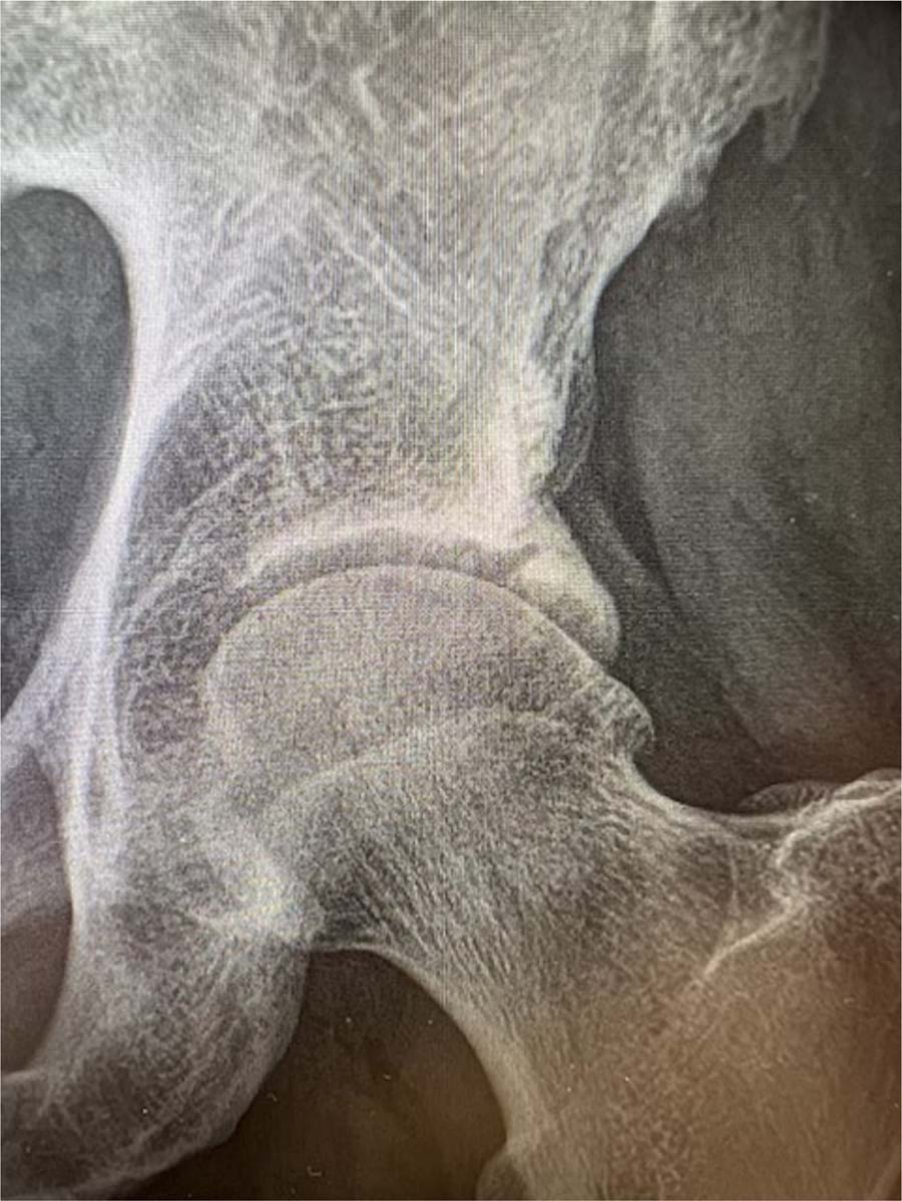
Radiographic image of ossified labrum.
